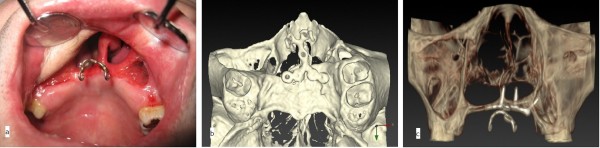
Postoperative view of miniplate fixation. (a) Frontal view of the titanium miniplate. (b) Occlusal view of a three-dimensional computed tomography (3D-CT) image with the titanium miniplate. (c) Frontal view of a 3D-CT image with the titanium miniplate.
